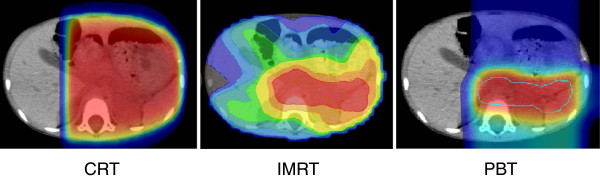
Dose distributions map of CRT, IMRT and PBT for representative case. Area irradiated with 95%(red), 70%(yellow), 40%(green) and 10%(blue) of prescribed dose were shown in CT image.
